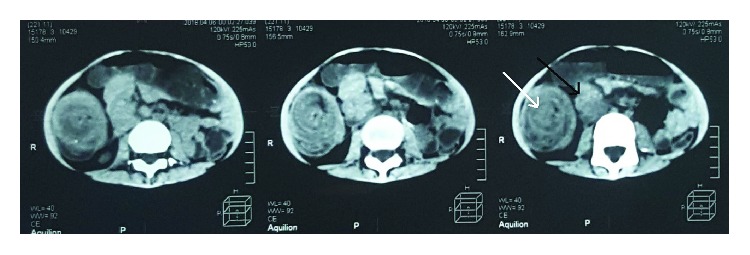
CT showing the “target” configuration (white arrow) and the mass (black arrow).
